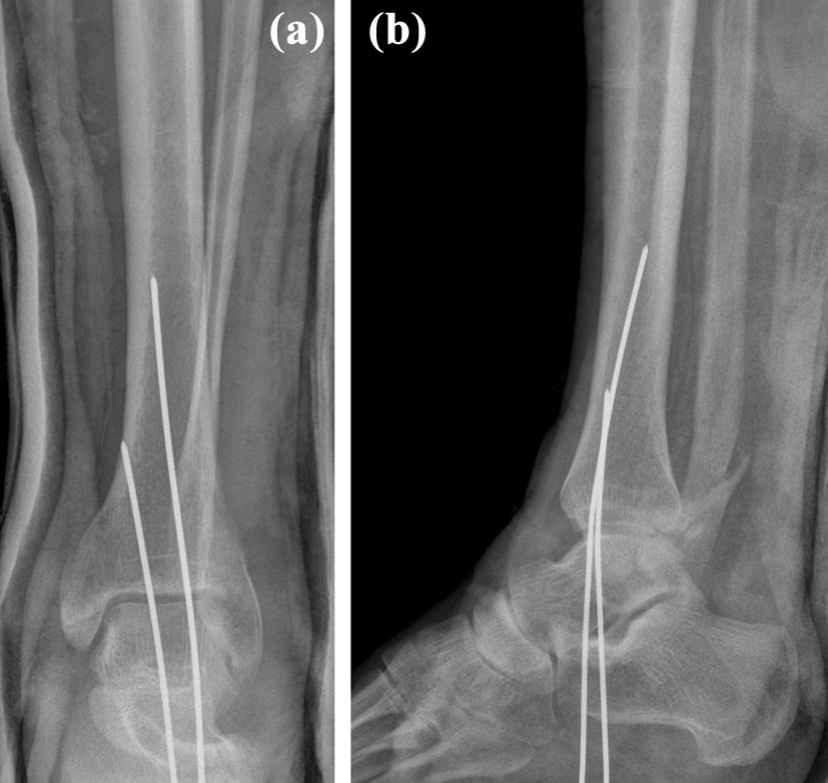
Radiograph after unsuccessful closed reduction under general anesthesia showing persisting a overlap of the distal tibia and fibula, and b tibiofibular dissociation
